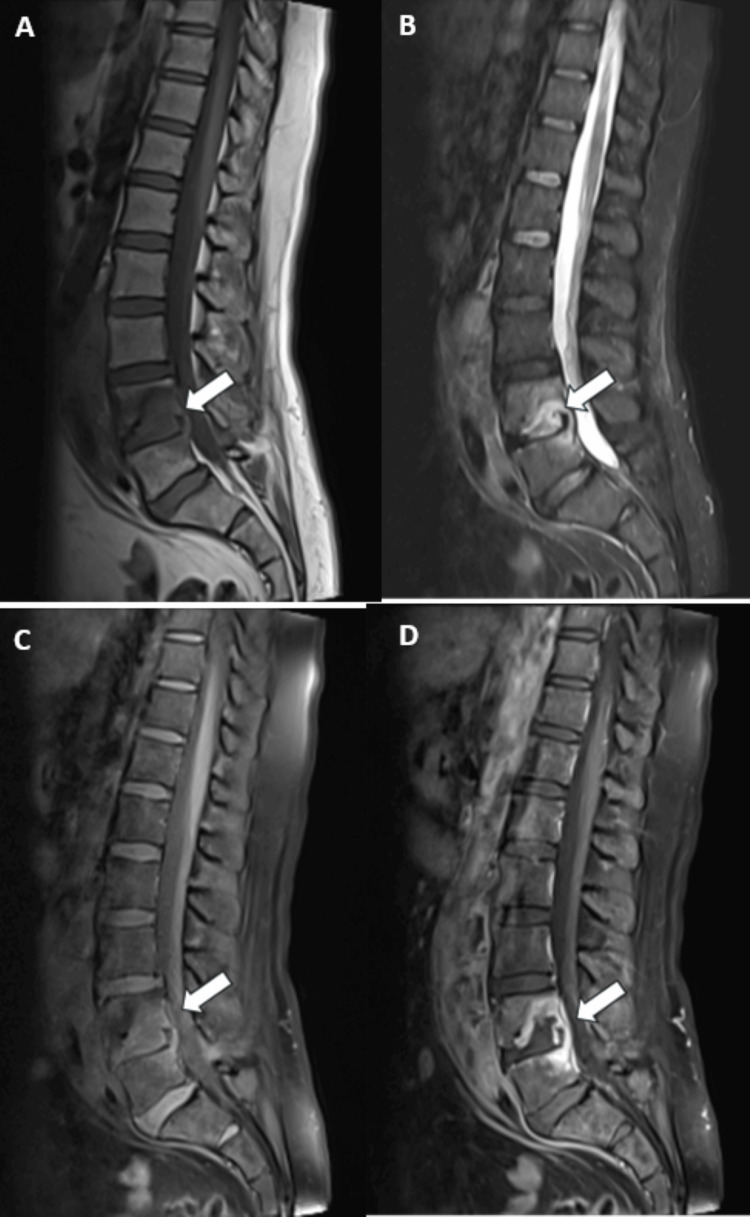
From left to right (A-D): sagittal T1, STIR, fat-suppressed T1 pre-contrast, and fat-suppressed T1 post-contrast images Destructive changes are seen in the posterior vertebral body and inferior endplate of the L4 vertebra, with a relatively preserved L4/L5 intervertebral disc (A-D). Oedematous changes - low signal on T1 (A) and high signal on STIR (B) - are present in the surrounding bone marrow of the L4 vertebral body, as well as along the posterior superior endplate of the L5 vertebra. The bony abnormality is associated with a multiloculated collection. The bony abnormality, as well as the psoas collection, shows heterogeneous peripheral enhancement on post-contrast fat-suppressed T1-weighted images. STIR, Short Tau Inversion Recovery
